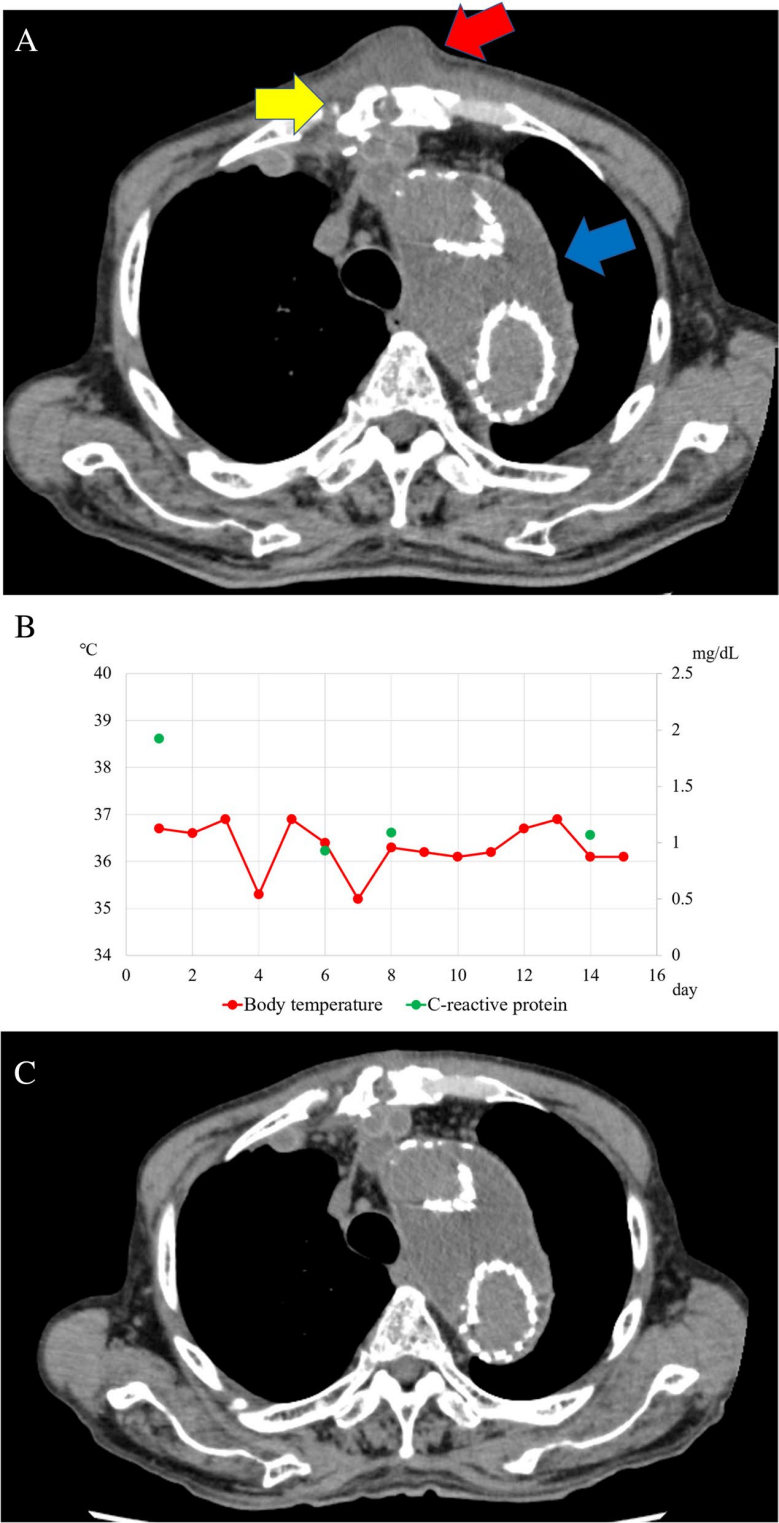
A CT findings in case 3. CT revealed a subcutaneous low-density mass (red arrow) sized 20 × 23 mm in the center of the median sternotomy, continuous with a 14-mm-wide area of fluid collection (blue arrow) around the ascending aortic graft. The gap of the closed sternum was also visualized (yellow arrow). B Chart for recording case 3 patient body temperature and C-reactive protein level. Shown is the chart of body temperature (red point) and C-reactive protein levels (green point) recorded during hospitalization. No fever was noted during hospitalization, and the C-reactive protein level remained stable without antibiotic therapy. C CT findings 1 month after initial treatment with aspiration drainage. The subcutaneous tumor had disappeared
